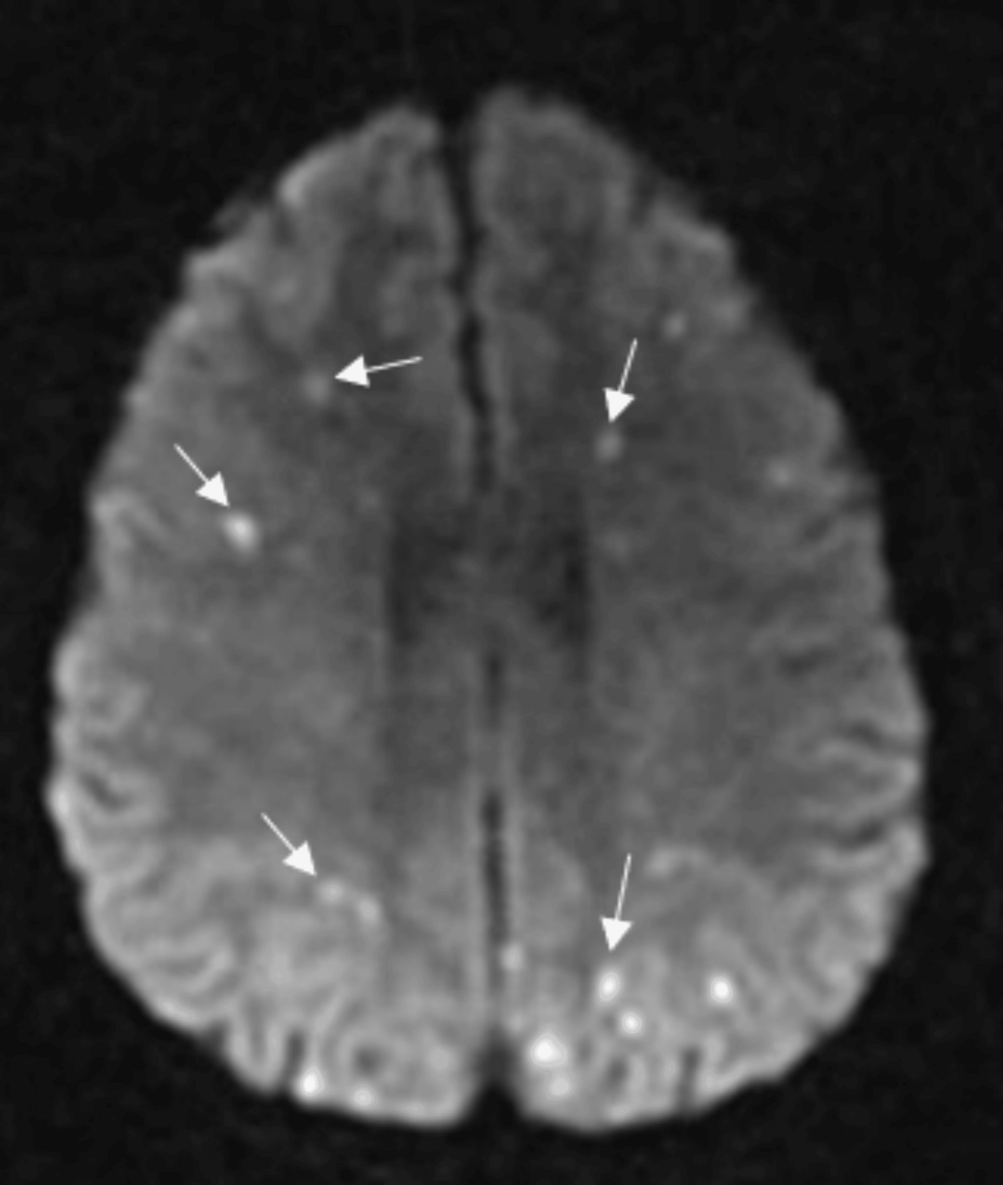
Diffusion-weighted magnetic resonance imaging of the brain This image shows evidence of acute vascular events, such as focal hyperintensities (white arrows). The bilateral distribution and involvement of multiple vascular regions suggest an embolic source.
